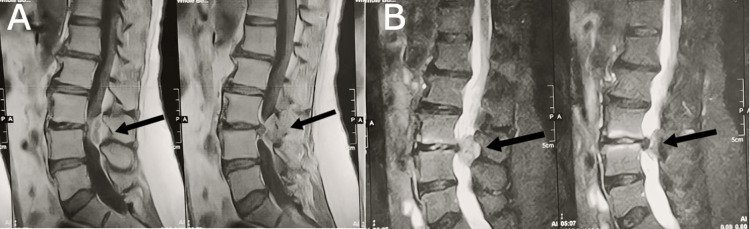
(A) Contrast-enhanced T1 MRI images of the lumbar spine show L3-4 disc prolapse associated with retrolisthesis. There is a dorsal, dural-based mass with an enhancing capsule. The core of the mass is non-enhancing. (B) T2-weighted images show the mass to be isointense. The patient presented with acute symptoms of cauda equina compression exacerbation. Based on this presentation, the decision was made to proceed with surgical excision of the mass. It was believed that the patient had two distinct pathologies: spinal instability and discogenic compression, which acutely worsened the chronic neoplastic compression. Although the mass is separated from the prolapsed disc by the enhancing capsule, the disc is in contact with the mass at the lateral area.
